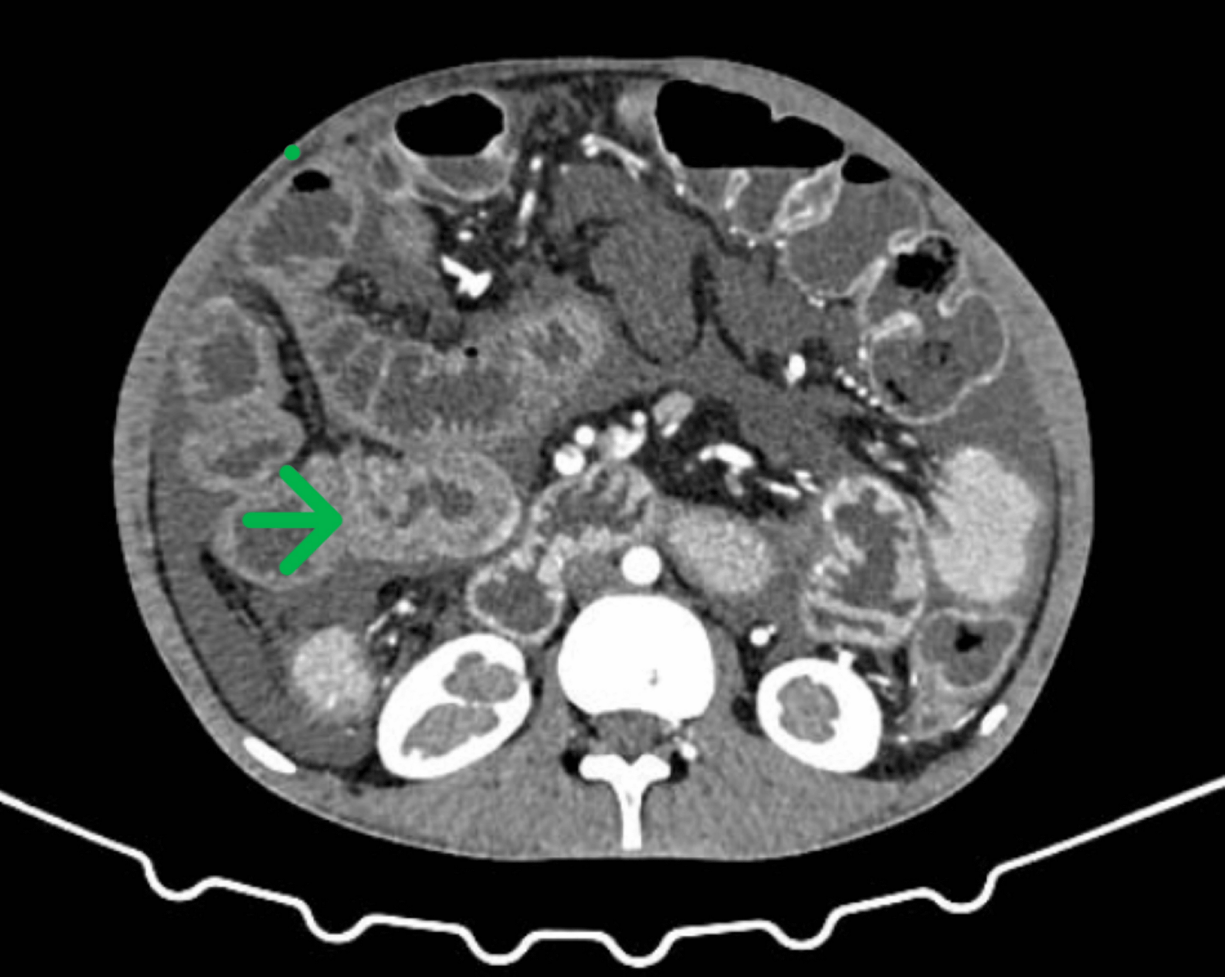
Triple-phase CT of the abdomen. Findings: Diffuse, circumferential, continuous, and long segmental, mural thickening was seen involving the ileal loops with a maximum thickness of 11 mm. Post-contrast imaging showed preserved mural stratification with mucosal and serosal enhancement and hypoenhancing submucosal edema was noted. No evidence of strictures/luminal narrowing was noted. No significant wall thickening was seen in large bowel loops. No obvious retroperitoneal lymphadenopathy. A severe amount of free fluid was seen involving perihepatic, hepatorenal, peri-splenic splenorenal, bilateral paracolic gutters, inter bowel spaces, pelvic spaces, and rectovesical pouch.
